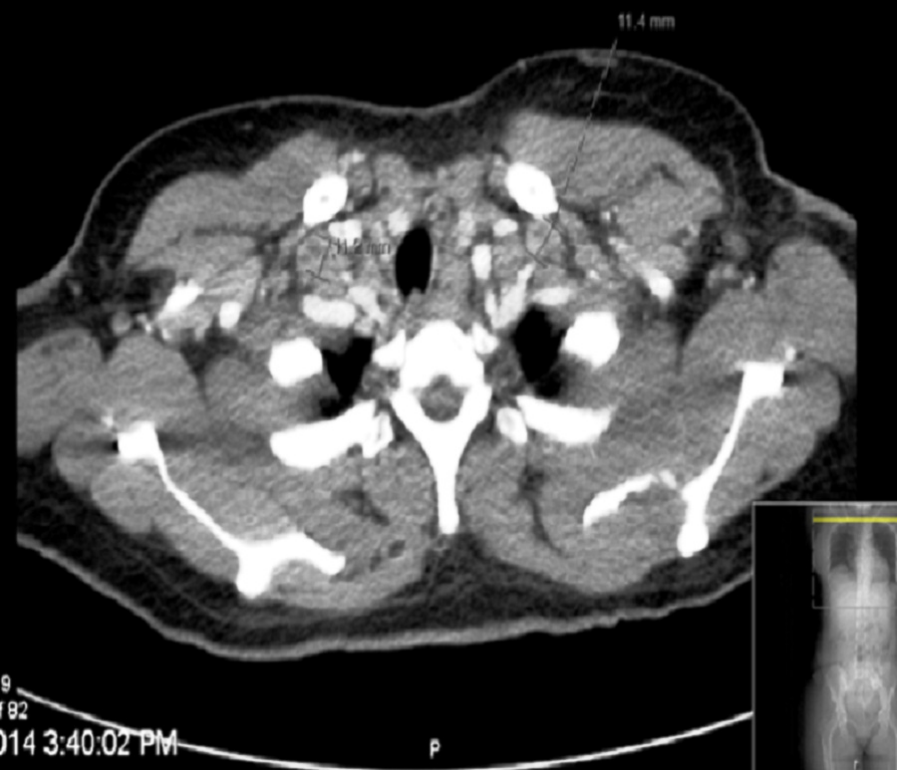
CT chest CT chest revealing right paratracheal, prevascular, subcarinal, and bilateral supraclavicular lymphadenopathy
